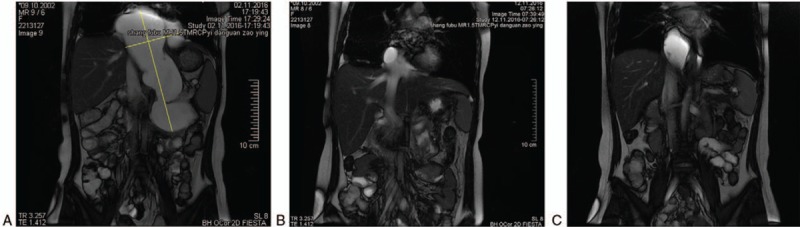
Radiographic examination at our center. (A) Preoperative MPCP revealed that the cystic lesion in the pancreas and the chest communicated via an esophageal hiatal hernia. (B, C) Postoperative MPCP showed reduced pleural effusion and a small cystic lesion in the right chest.
